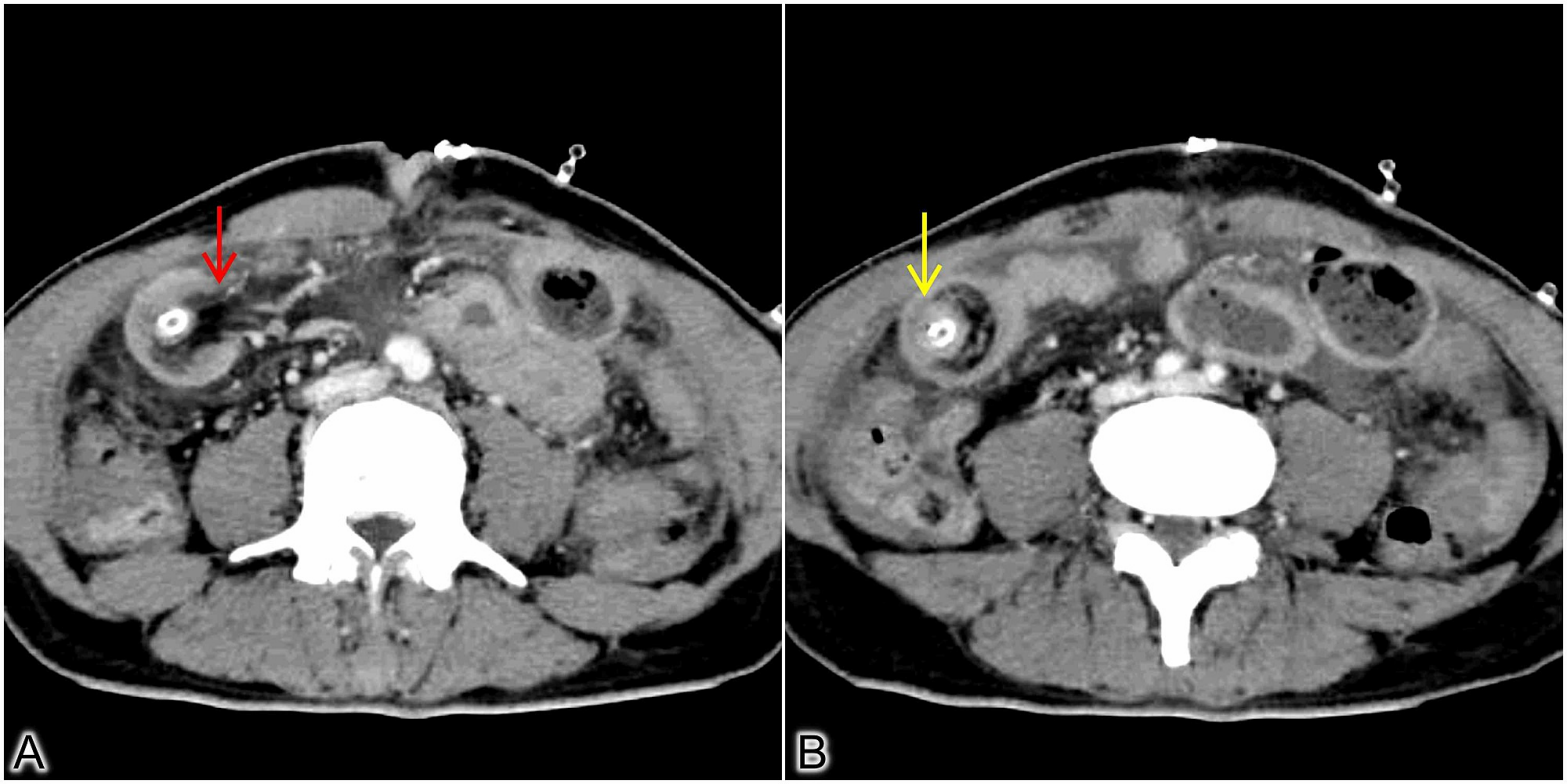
CECT images. (A) Axial section at the region of the start of intussusception. A bowel loop with its mesentery is seen entering into the adjacent bowel lumen with the tube in situ (red arrow). (B) Axial section at the mid of the intussusception showing the bowel within bowel appearance (yellow arrow). Bowel loops are seen well enhancing, indicating the intact vascularity. CECT - Contrast-Enhanced Computed Tomography
